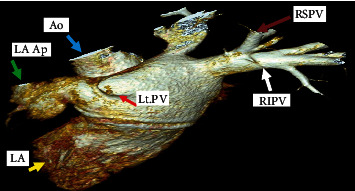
Three-dimensional cardiac CT reconstruction images: absent of left pulmonary veins following AF ablation (red arrow). CT: computed tomography; Ao: aorta (blue arrow); LA Ap: left atrial appendage (green arrow); LA: left atrium (yellow arrow); RSPV: right superior pulmonary vein (brown arrow); RIPV: right inferior pulmonary vein (white arrow); AF: atrial fibrillation.
